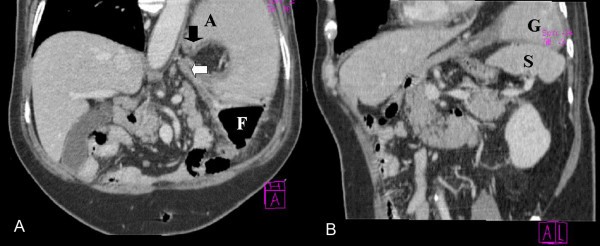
(A) Coronal reconstructed computed tomography images showing a rotated, 'right-side up' position of the stomach with the pylorus (black arrow) superior to the cardio-oesophageal junction (white arrow). The fundus (F) is inferior and the antrum (A), superior. (B) Spleen (S) displaced inferior to the gastric body (G).
